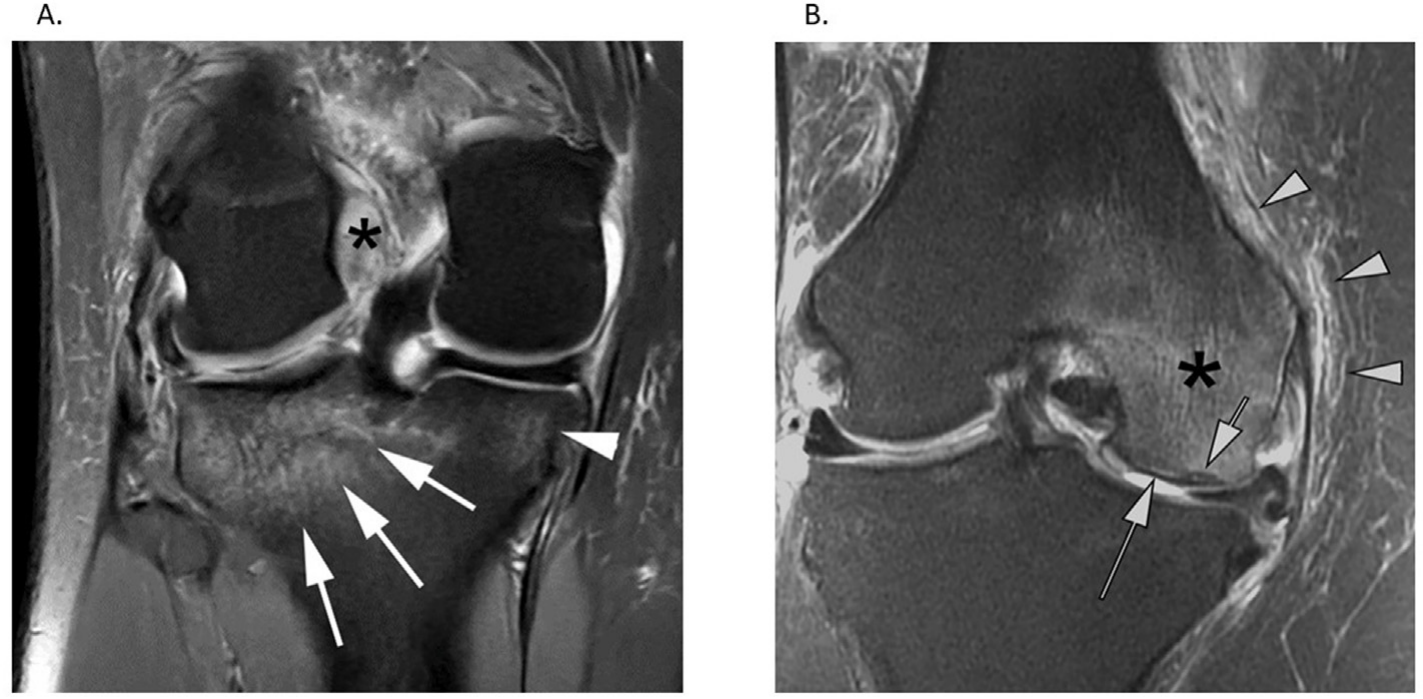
Bone marrow lesions caused by alternative pathologies to OA. A. BML caused by trauma: Coronal fat-suppressed intermediate-weighted MRI shows hyperintensity of the posterior lateral tibial plateau, but there is no fracture line (arrows). There is also a smaller hyperintense lesion is visible at the posterior medial tibia (arrowhead). There is also a traumatic anterior cruciate ligament tear (asterisk) and the bone marrow changes are consistent with bone contusions found in association with the cruciate ligament tear. B. BML caused by Subchondral Insufficiency Fracture (SIF). Coronal fat-suppressed MRI shows a subchondral linear hypo-intensity zone directly adjacent to the normal subchondral plate (short arrow) at the medial femoral condyle. There is also extensive bone marrow hyperintensity of the femoral condyle (‘bone marrow oedema’, asterisk) and soft tissue hyperintensity (‘inflammation’) at the medial joint line (arrowheads). Subchondral linear hypo-intensity is pathognomonic for SIF. There is also full-thickness cartilage loss at the central medial femur (long arrow) and meniscal extrusion due to a posterior medial meniscus root tear, which are commonly found with SIF.
